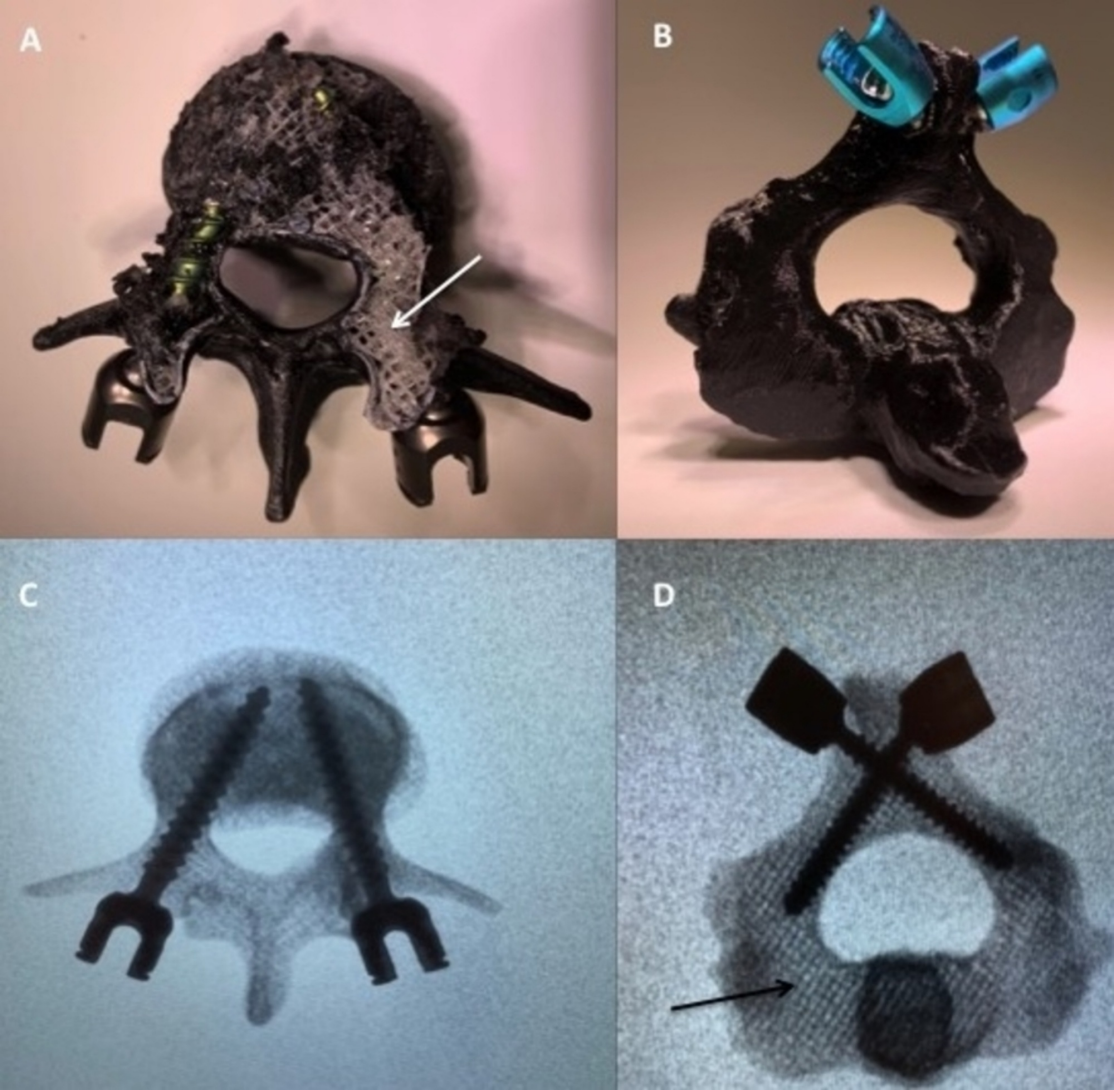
Instrumentation examples of experimental (PLA/PVA) models (A) L3 vertebrae with PLA shell removed demonstrating the spongy PVA matrix (white arrow). Pedicle screws are seen within the matrix. (B) Laminar screws placed in the C2 experimental model without model failure. (C & D) Fluoroscopic images obtained post instrumentation. The PVA infill matrix can be seen under X-ray (black arrow). PLA, polylactic acid; PVA, polyvinyl alcohol
